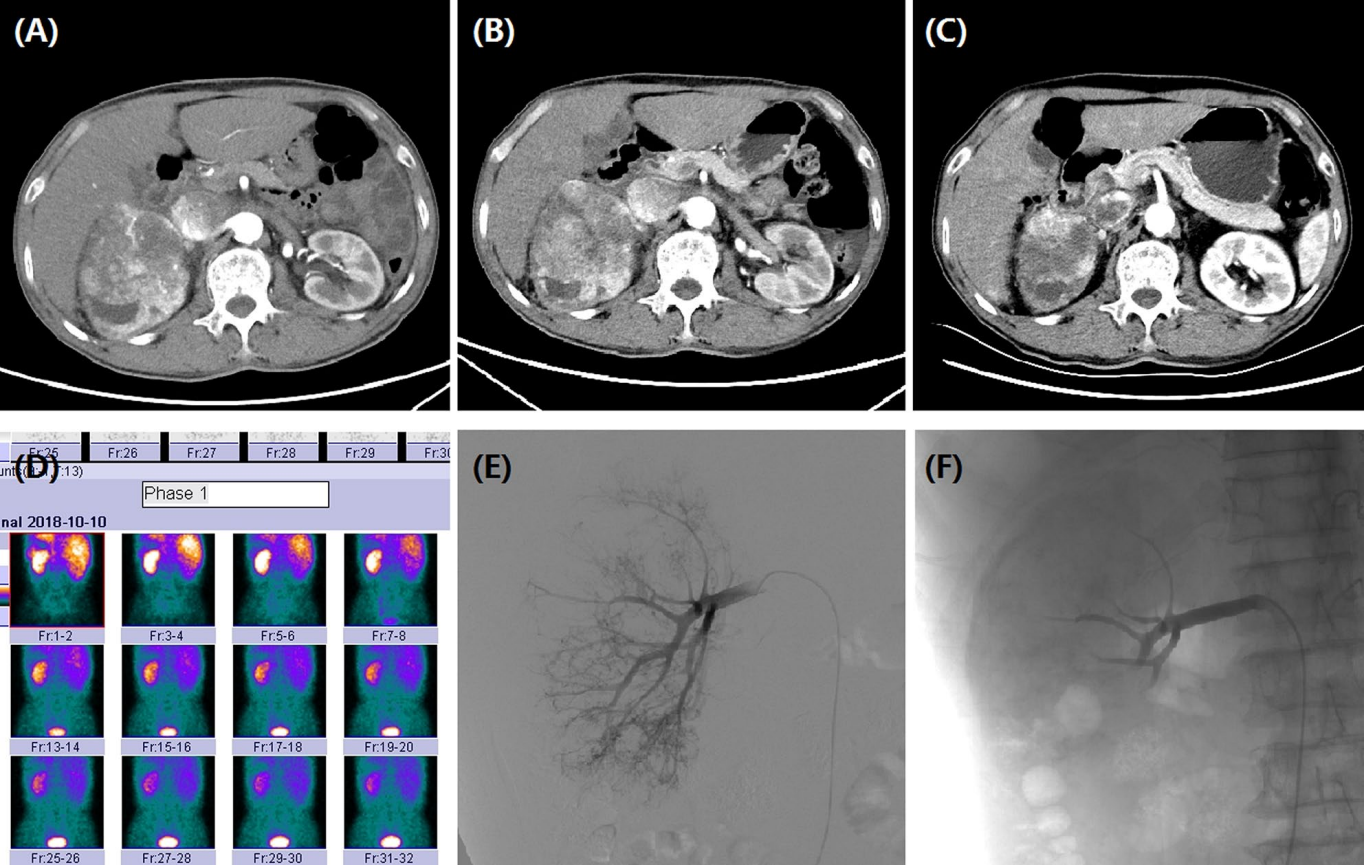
A 72-year male with right RCC treated by CB. (A) CT preoperative examination revealed a large tumor of the right kidney with tumor invasion into inferior vena cava. (B) The right renal tumor maintained stable after one month's follow-up. (C) The tumor shrunk at 3 months after DEB-TACE. (D) The right kidney showed a lower GFR (43.7 ml/min) than the left kidney (60.1 ml/min). (E) The right renal artery was the blood supply artery of the tumor. (F) The right renal artery was incubated and embolized.
